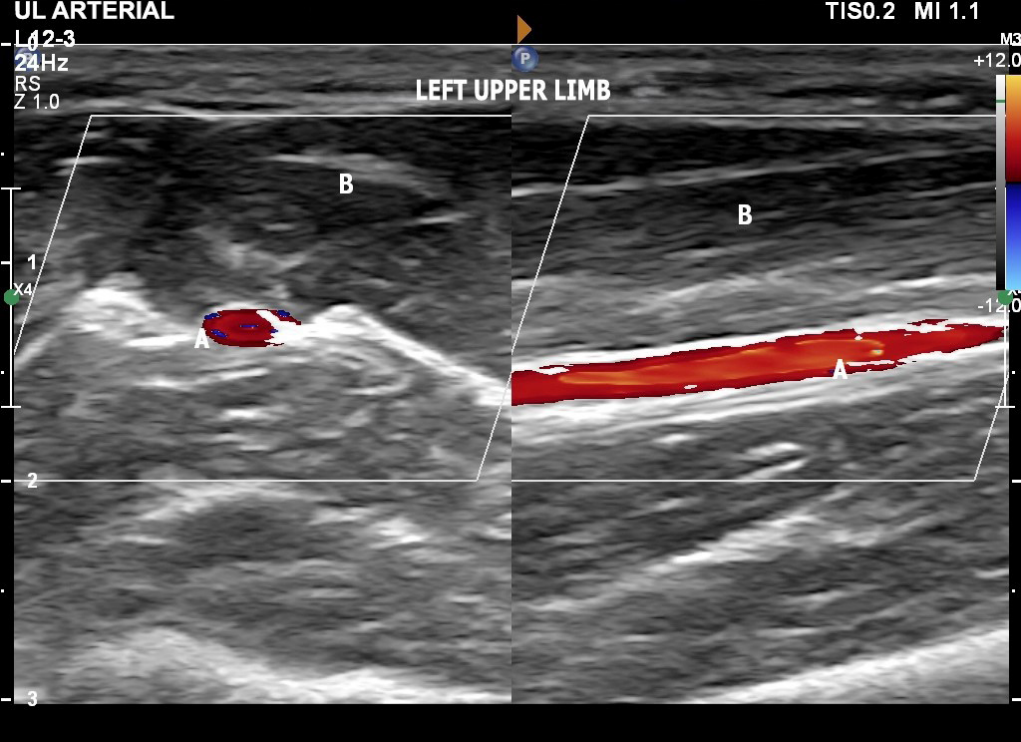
Doppler ultrasound of the left upper limb with the ulnar artery in its normal position, showing the transverse image on the left and the longitudinal image on the right. Labels indicate (A) the ulnar artery, in a superficial position, and (B) the flexor muscles of the forearm.
